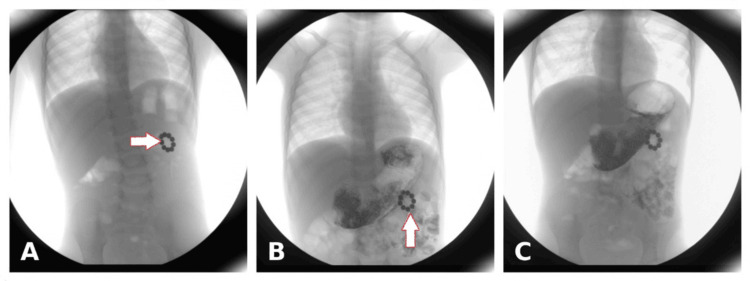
Barium meal X-ray of stomach and duodenum. (A) and (B) Barium meal X-ray of the stomach shows a circular metallic foreign body with a beaded appearance (white arrow) at the greater curvature of the stomach. (C) The duodenal cap appears normal in shape and is well opacified with contrast.
